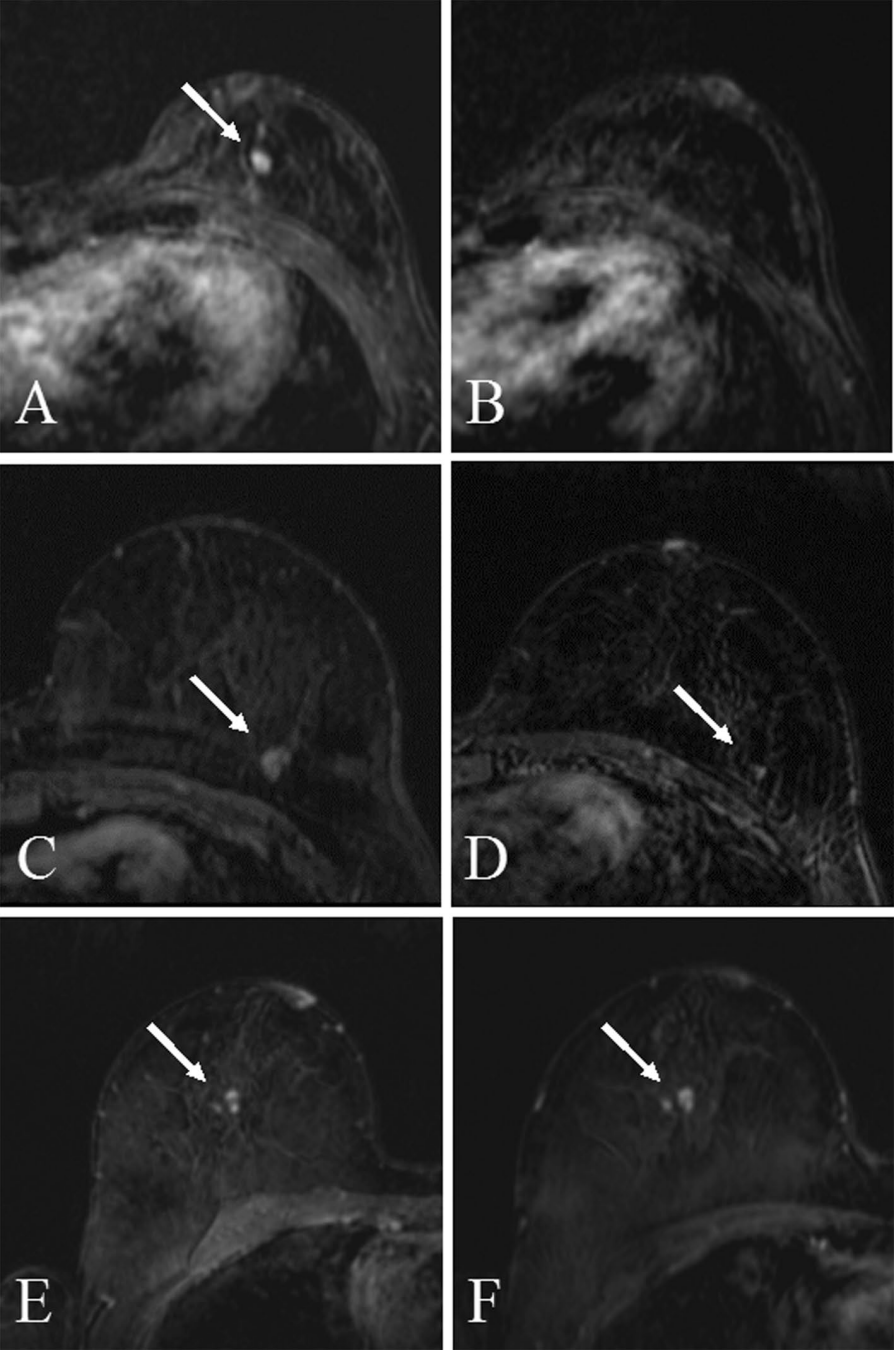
First the subtraction images of a breast cancer invasive ductal carcinoma (IDC) grade 3 (a), which were rated as invisible in the prior MRI (b), second row are the images (c) rated as minimal sign in the prior image (d) also showing an IDC grade 3, and the last row are the images of the current MRI (e) and the visible lesion in the prior MRI (f) an invasive lobular carcinoma grade 2
